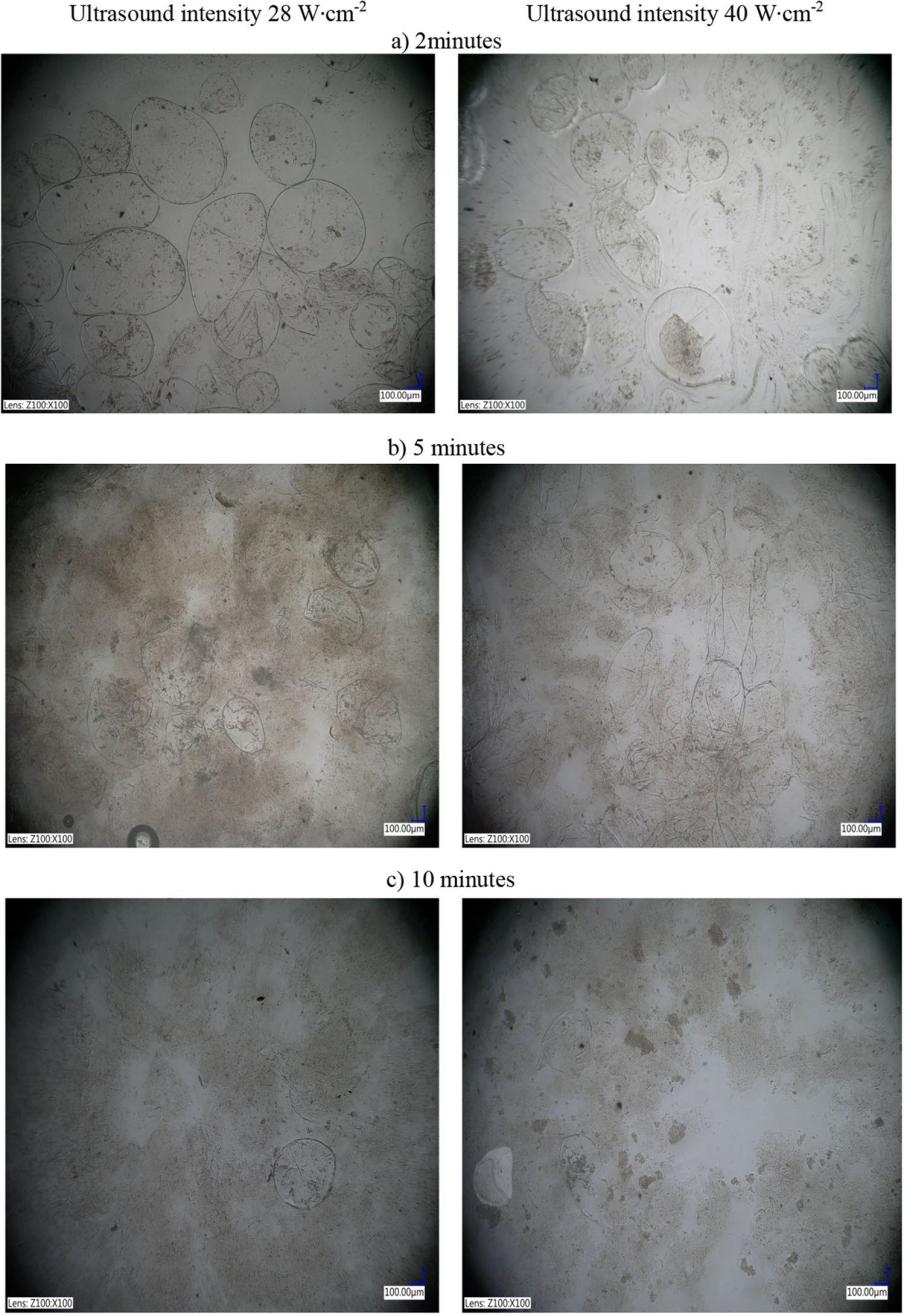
Microstructure of tomato juice sonicated at ultrasound intensity of 28 and 40 W cm^−2^ for: (a) 2 min, (b) 5 min, and (c) 10 min.
